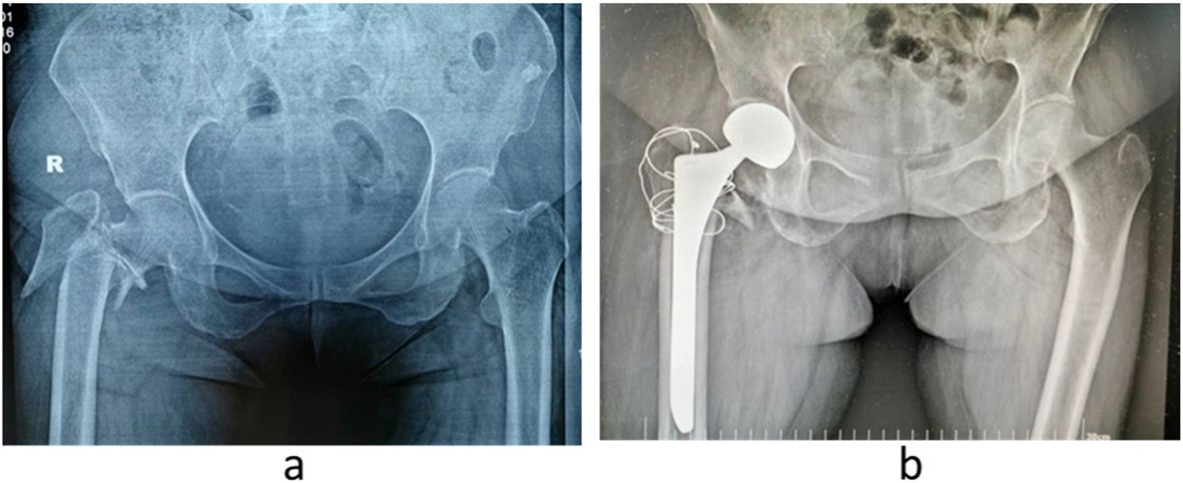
a Anteroposterior radiograph showing an unstable intertrochanteric fracture of right hip in a 82-year-old male patient who fell at home. b Radiograph one year after hemiarthroplasty
